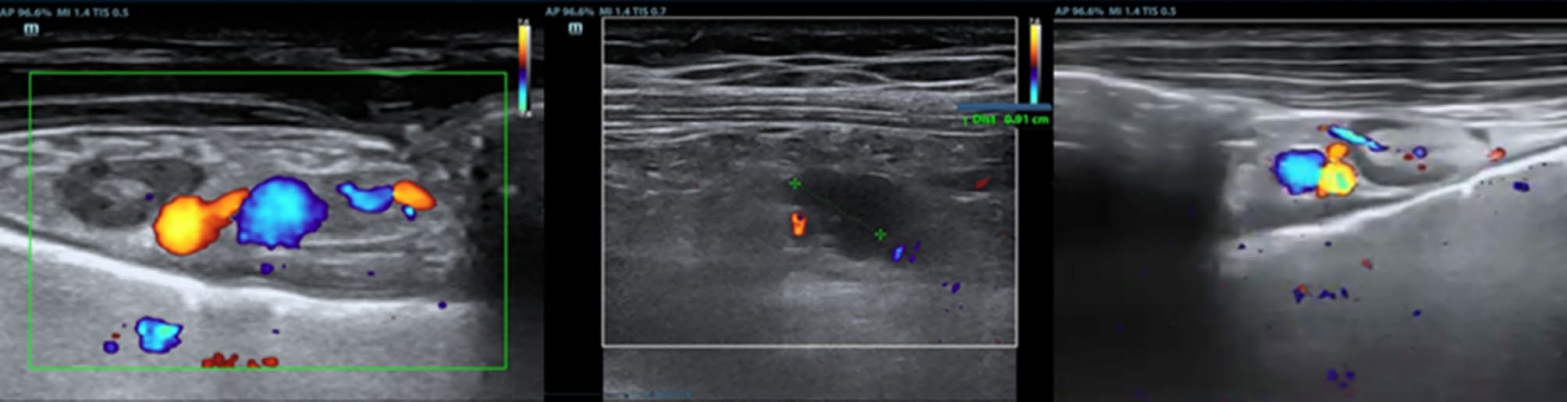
The IMLN is more frequently visualized at the second and third intercostal space on the parasternal line, lateral to the internal mammary vessels (a), between the artery and the vein (b) or medial (c)
